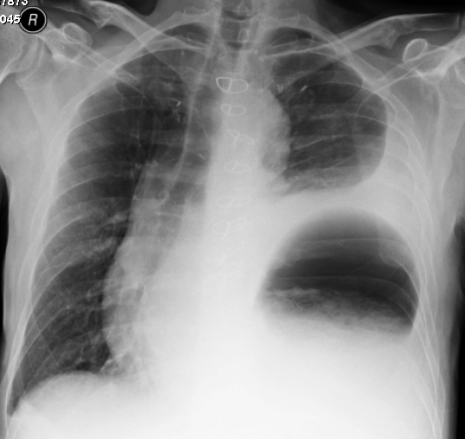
X-ray of the thorax with suspected intrathoracic stomach.
